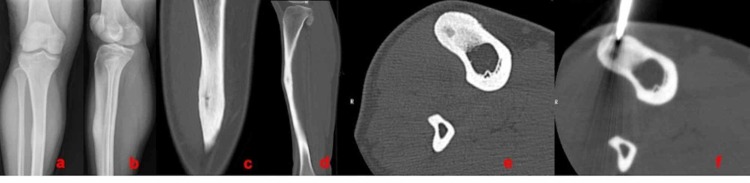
A 20-year-old female patient with OO localized in the a) tibia; b) AP and lateral X-ray; c), d), and e) coronal, sagittal, and axial CT view; and f) axial CT view during the RFA procedure OO: osteoid osteoma; AP: anteroposterior; CT: computed tomography; RFA: radiofrequency ablation
